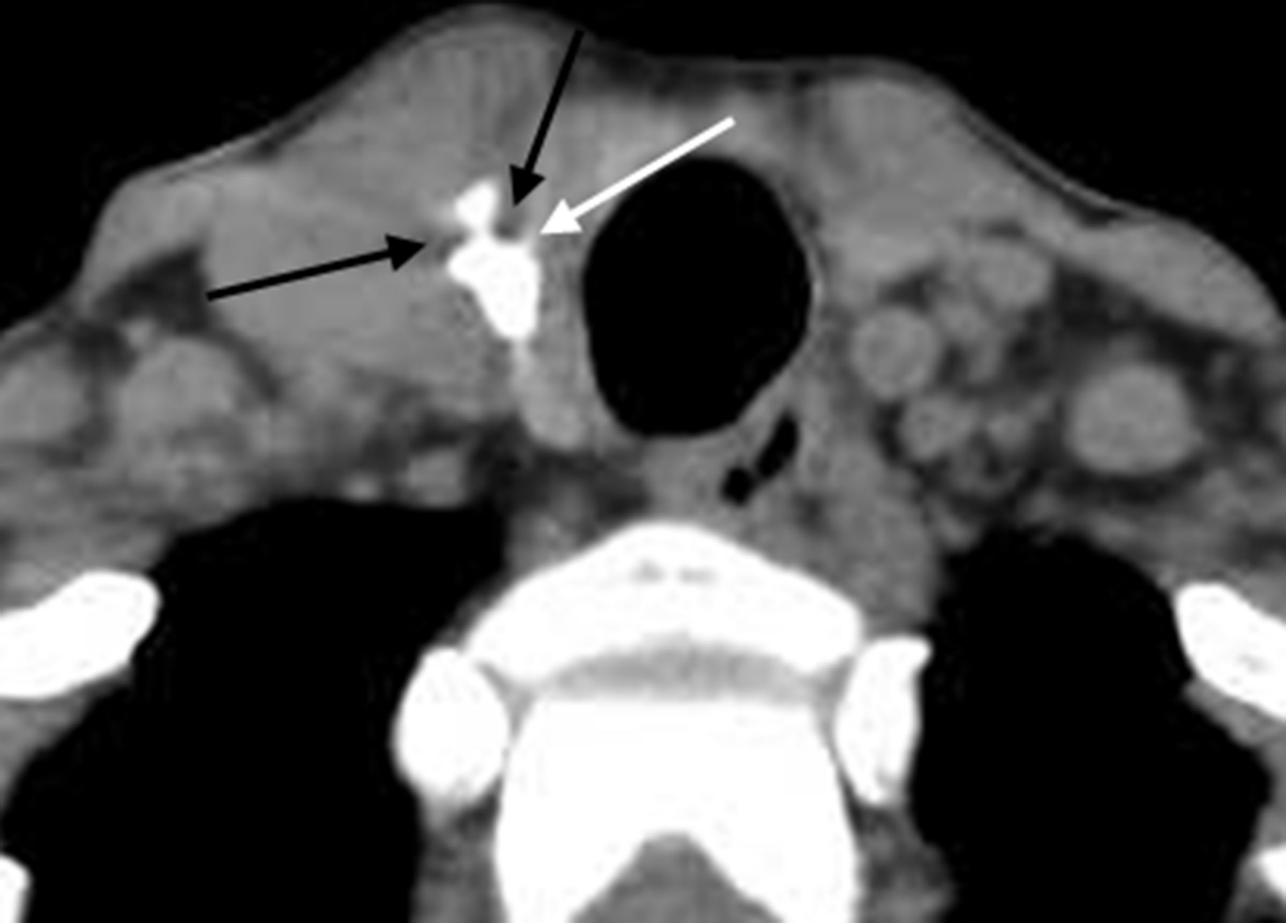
Unenhanced CT showed obvious cupping artifacts (white and black arrow). The lesion was pathologically confirmed as NG.
